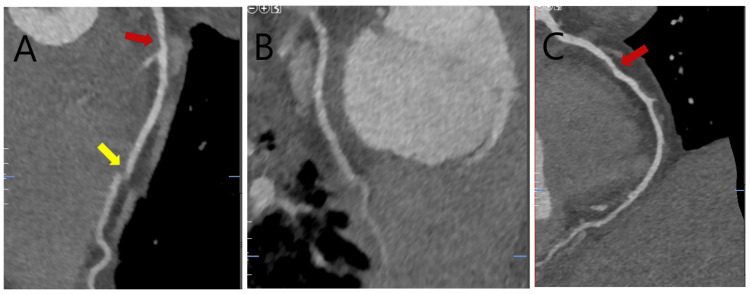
Curved multiplanar reformatted multidetectory coronary CT angiography images LAD: Left anterior descending artery; RCA: Right coronary artery Assessment of some segments of the coronary arteries was limited by motion and stitch artifacts. The LAD (image A) contained a region of non-calcified plaque with mild (less than 50%) luminal stenosis in the mid-segment (red arrow). A segment of the distal LAD was not well evaluated due to stitch artifact (yellow arrow). The evaluable segments of the left circumflex coronary artery (image B) revealed no obstructive coronary artery disease. The right coronary artery (image C) contained non-calcified plaque in the mid-segment with mild (less than 50%) luminal stenosis (red arrow).
